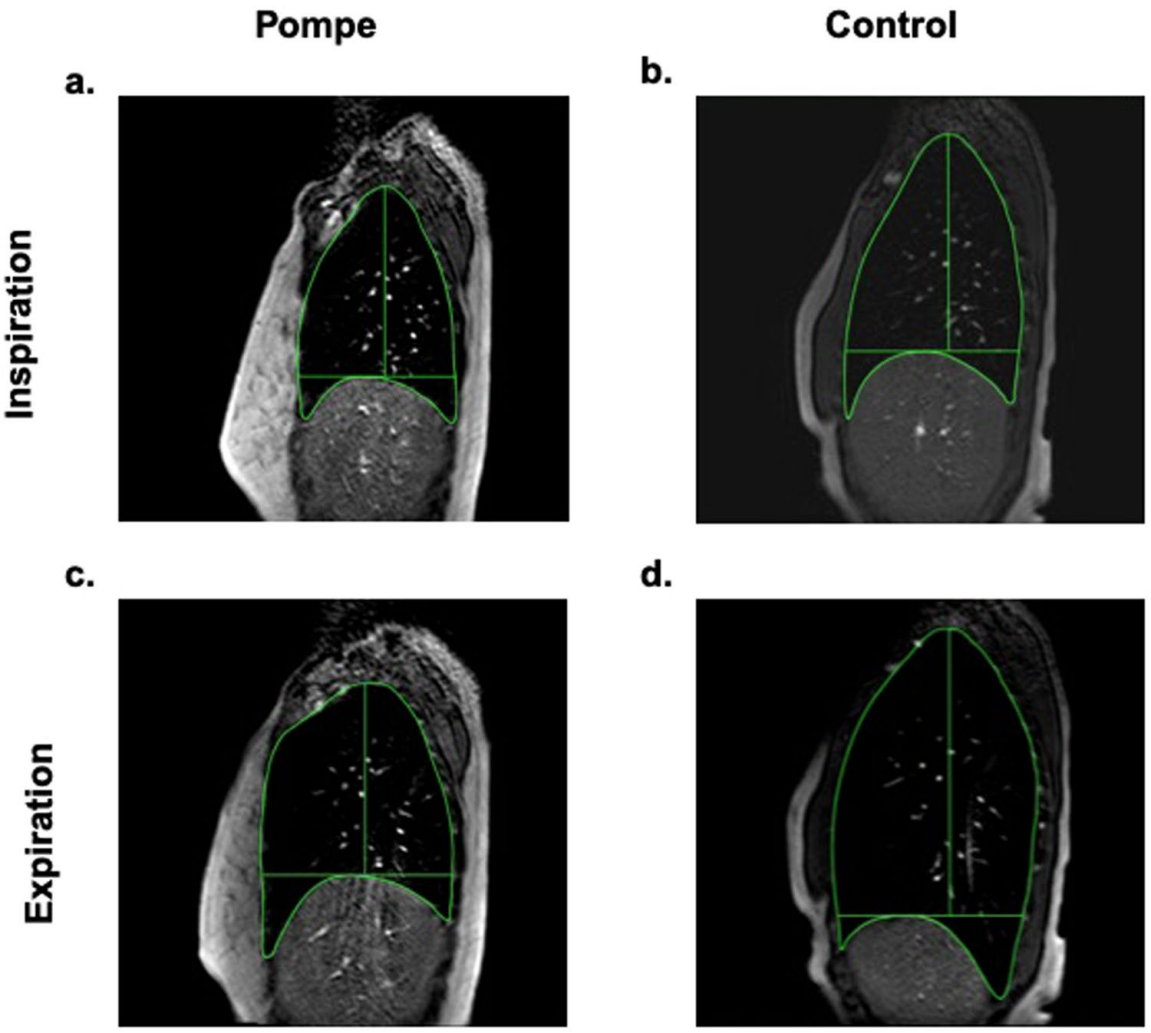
Right sagittal thoracic MRI images at end-inspiration and end-expiration during an inspiratory capacity maneuver. At end-exhalation, lung volumes were similar in (a) subjects with late-onset Pompe disease (subject 3 shown) and in (b) healthy controls (subject 14 shown). (c) In Pompe disease, maximal inspiratory volume generation occurred exclusively through anterior-posterior (A-P) expansion of the chest wall. In contrast, (d) control subject generated volume primarily through diaphragm descent, measured as increased cranio-caudal (C-C) distance.
